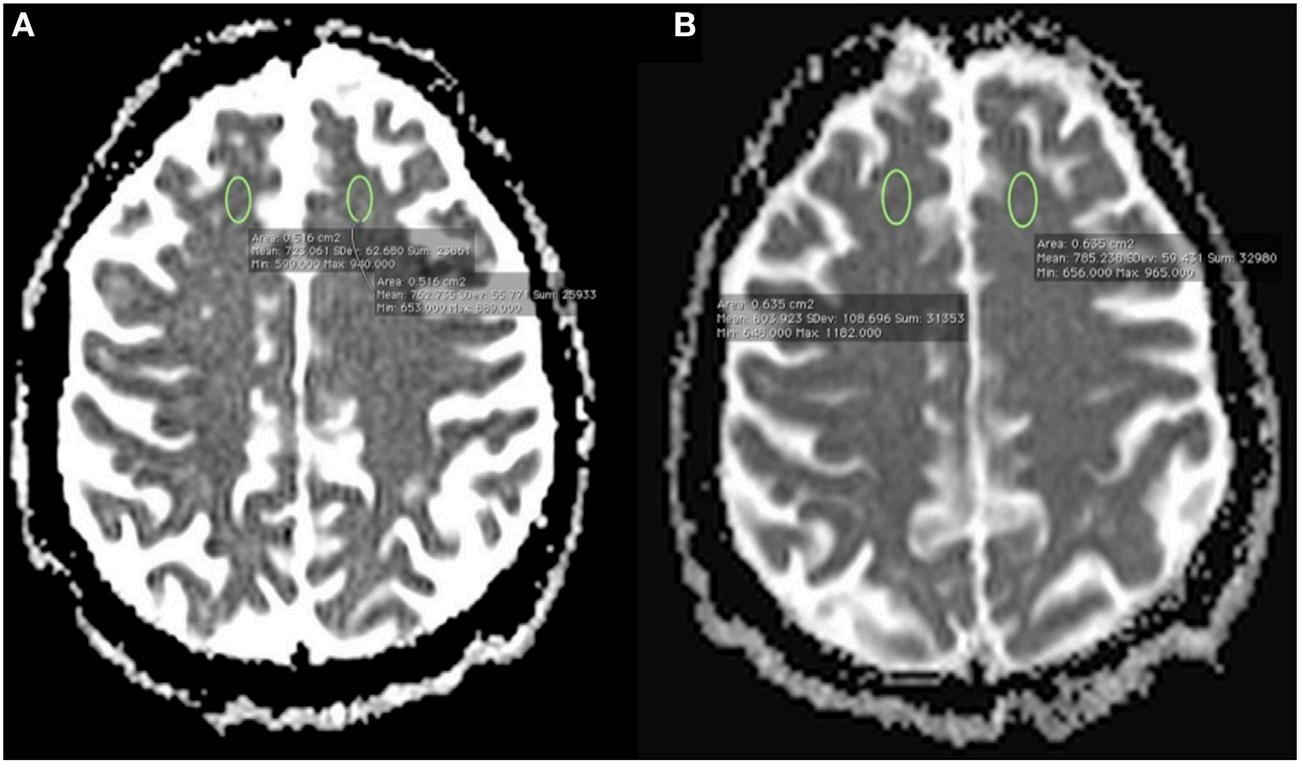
(A) 1.5 T brain magnetic resonance imaging (MRI) performed on day 1 showing a right dorsolateral prefrontal cortex (rDLPFC) hyperintensity on diffusion-weighted imaging with a significant decreased apparent diffusion coefficient (ADC) as compared to the homotopic region in the left hemisphere [difference of 0.039 × 10^−3^ mm^2^/s, showing 0.723 in the rDLPFC versus 0.762 in the left DLPFC (×10^−3^ mm^2^/s)]; (B) control brain MRI performed on day 9 after the onset of symptoms using the same MRI scanner and identical acquisition parameters revealing normal ADC levels on both DLPFC areas [no significant difference, showing 0.803 in the rDLPFC versus 0.785 in the left DLPFC (×10^−3^ mm^2^/s)].
